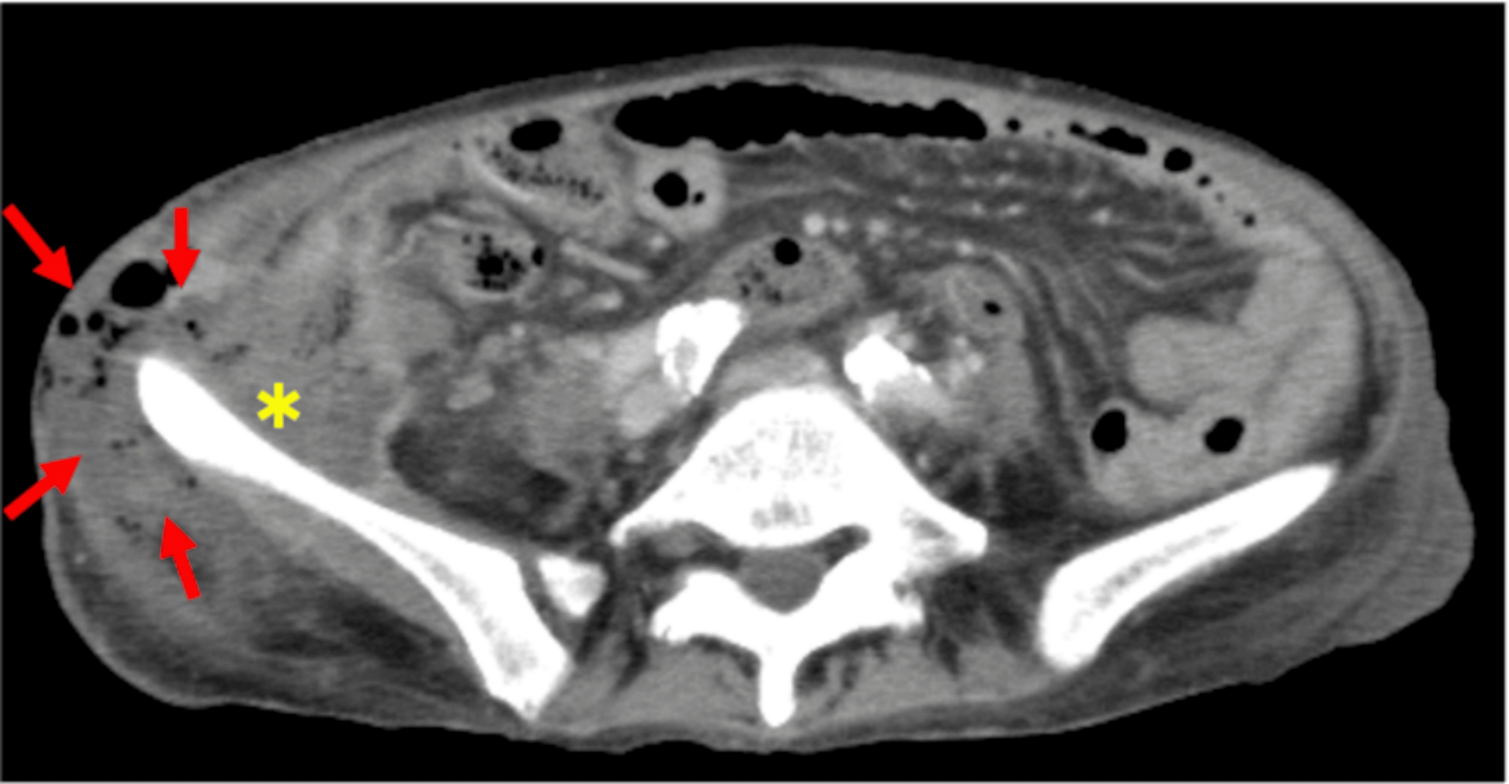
Computed tomography image at initial presentation An abscess is observed on the anterior aspect of the ilium (*) with a fistulous tract extending to the skin (→)
